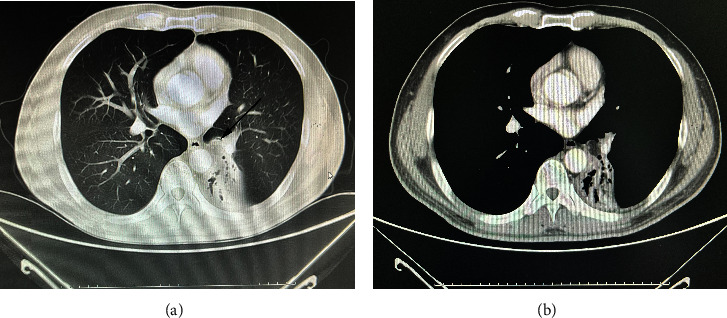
The findings of the endobronchial lipoma on chest CT showing a low attenuation lesion in the proximal bronchus of the left lower lobe on the lung (a) and mediastinal window (b).
